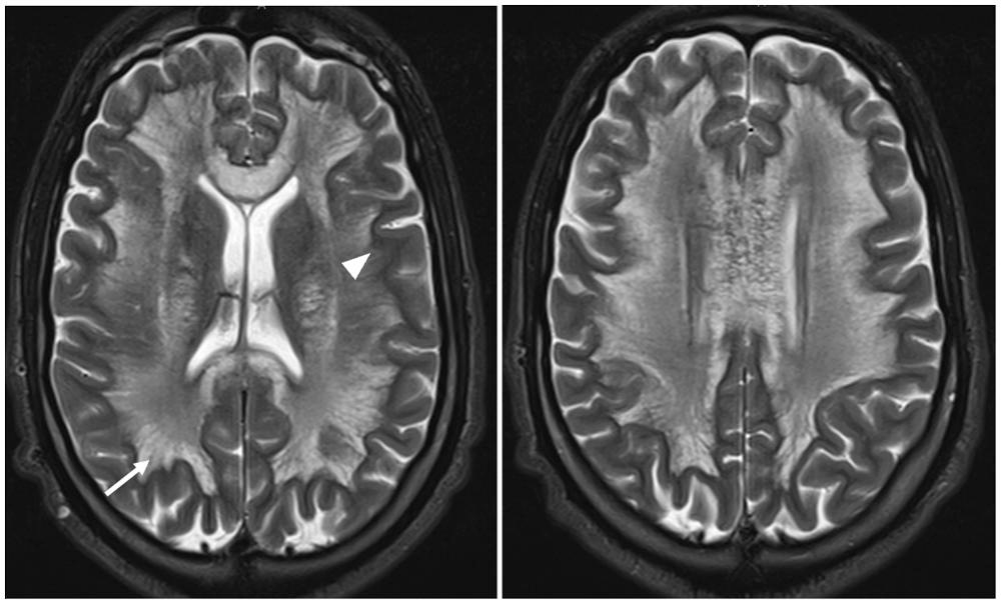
Axial T2-weighted slices depict diffuse bilateral white matter lesions respecting the U fibres (arrowhead) and exhibiting the characteristic “spongiform” appearance described in HLE, this refers to the visualization of vacuoles with a quasi-liquid signal located between the nerve fibres (arrow). Abbreviation: HLE = heroin-induced leukoencephalopathy.
